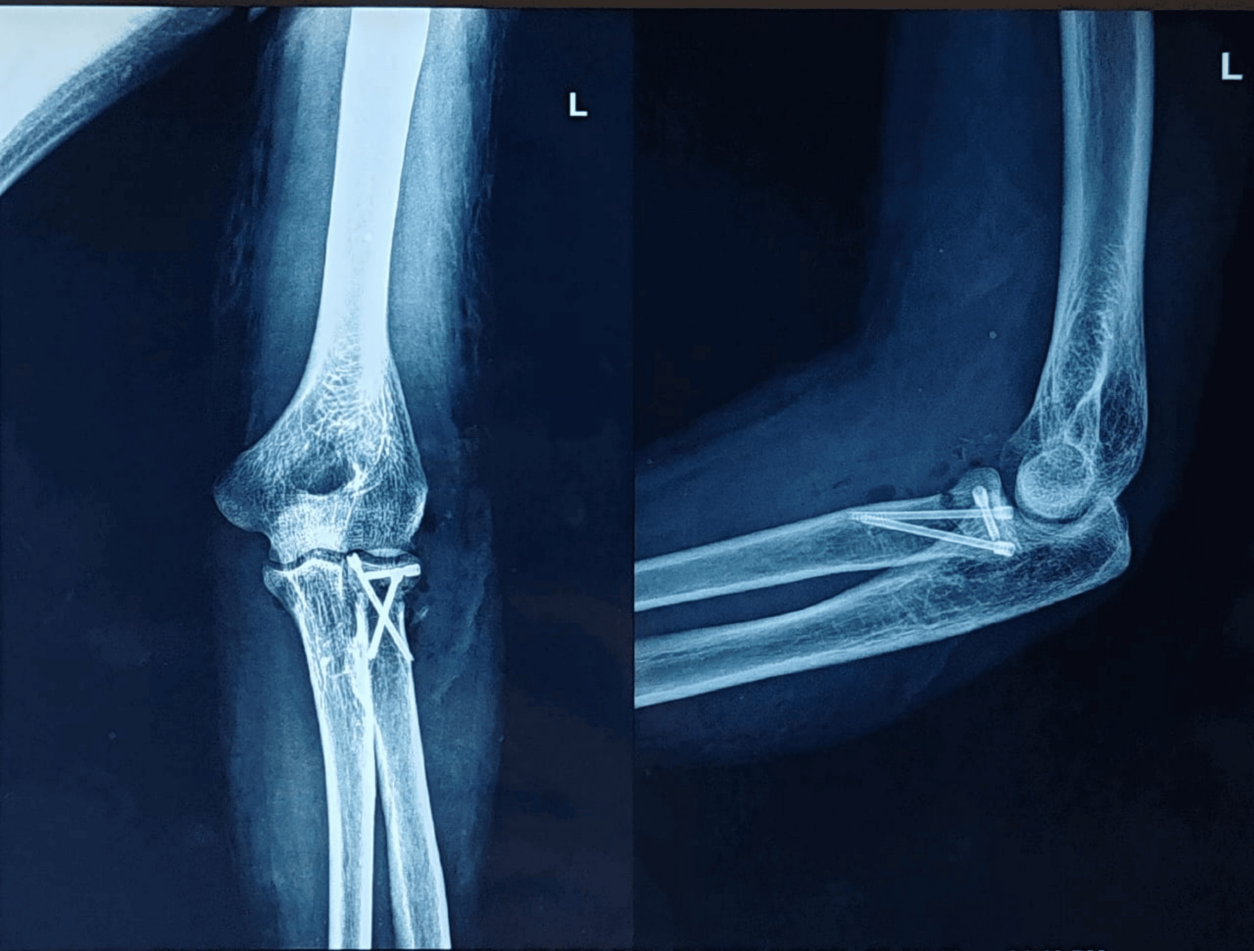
Immediate postop X-ray showing AP and lateral views of the left elbow joint with the radial head fracture reduced with the help of three headless compression screws AP: Anteroposterior
